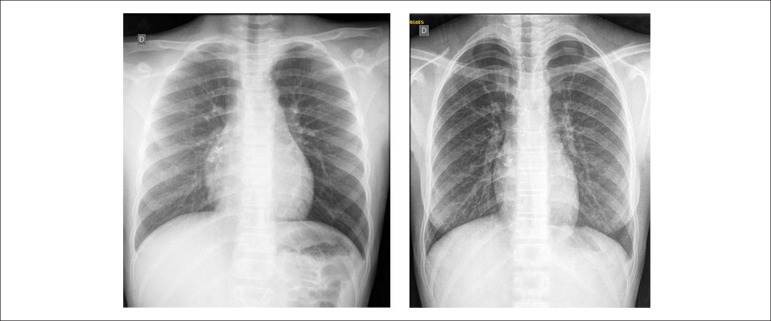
Chest X-rays before (left) and 5 years after (right) coronary-cavitary
